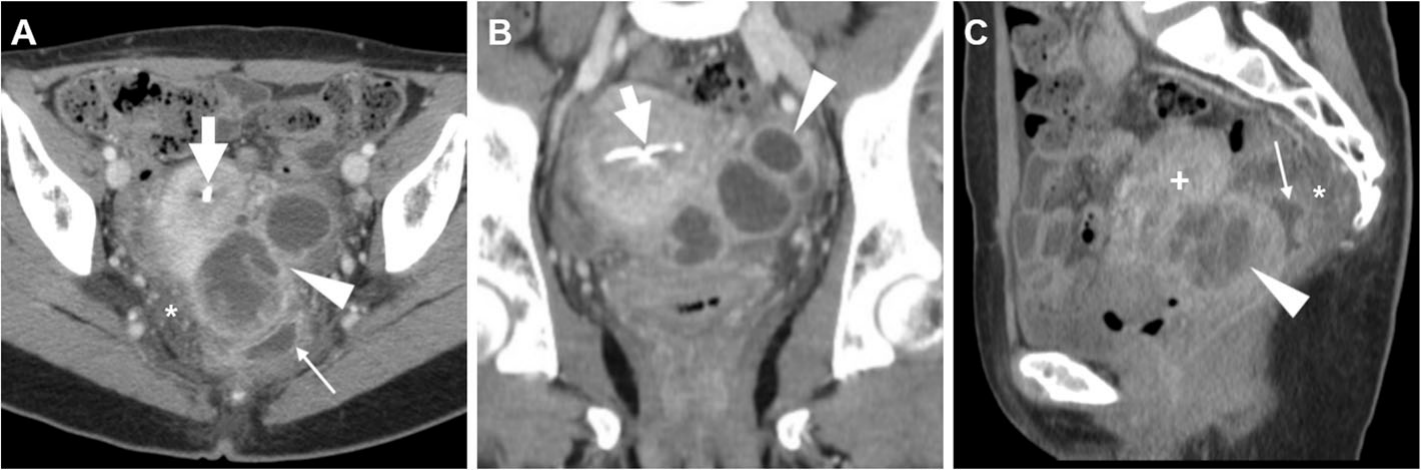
a–c Multiplanar CT images of a complex, septated left-sided TOA (arrowheads) in a 42-year-old woman, with characteristic irregular peripheral enhancement, that displaces the retroverted uterus (plus sign). Note metallic intrauterine contraceptive device (IUCD, thick arrows), minimal peritonitis of the cul-de-sac (thin arrows) and inflammatory stranding of the presacral fat (asterisk). The IUCD had to be removed
